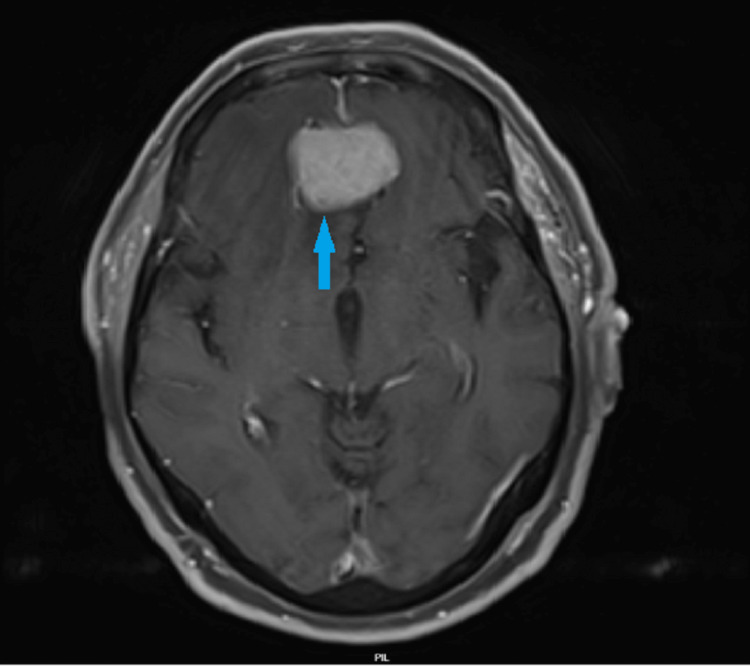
Brain MRI showing extra-axial dural-based solid mass suggestive of meningioma Brain MRI (T1-weighted imaging) showing an avidly enhancing extra-axial dural-based solid mass lesion (3.8×3.5×2.5 cm) in the anterior-inferior aspect of frontal lobes in the midline just above the cribriform plate (olfactory groove) suggestive of meningioma (blue arrow). MRI, magnetic resonance imaging
